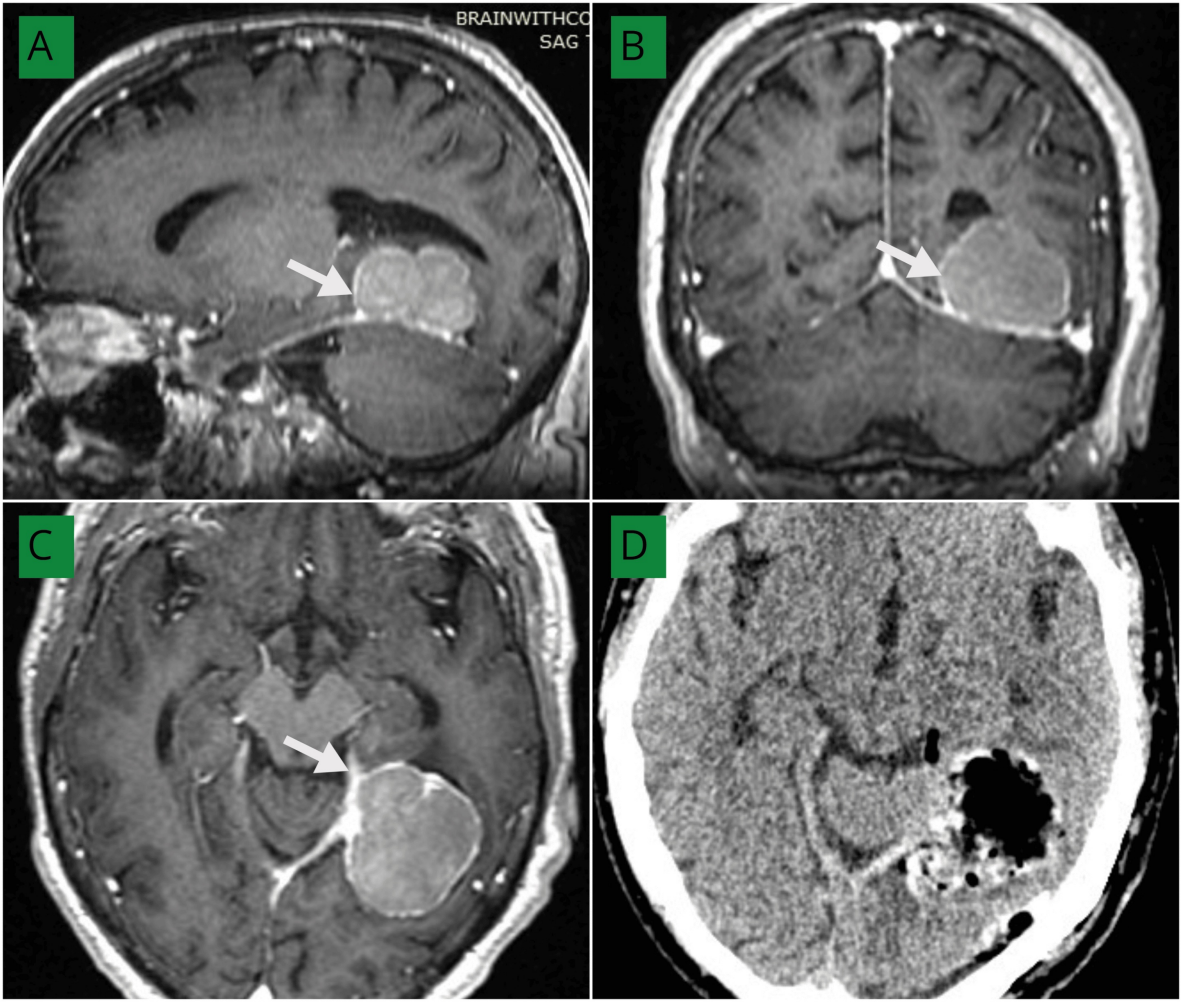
(A–C) Post-contrast MRI brain—axial (C), sagittal (A), and coronal (B) views demonstrating a homogeneously enhancing extra-axial mass attached to the left tentorium cerebelli and extending superiorly (white arrows). (D) Postoperative non-contrast CT brain (axial view) showing complete excision of the tumor. MRI: Magnetic resonance imaging, CT: Computed tomography
